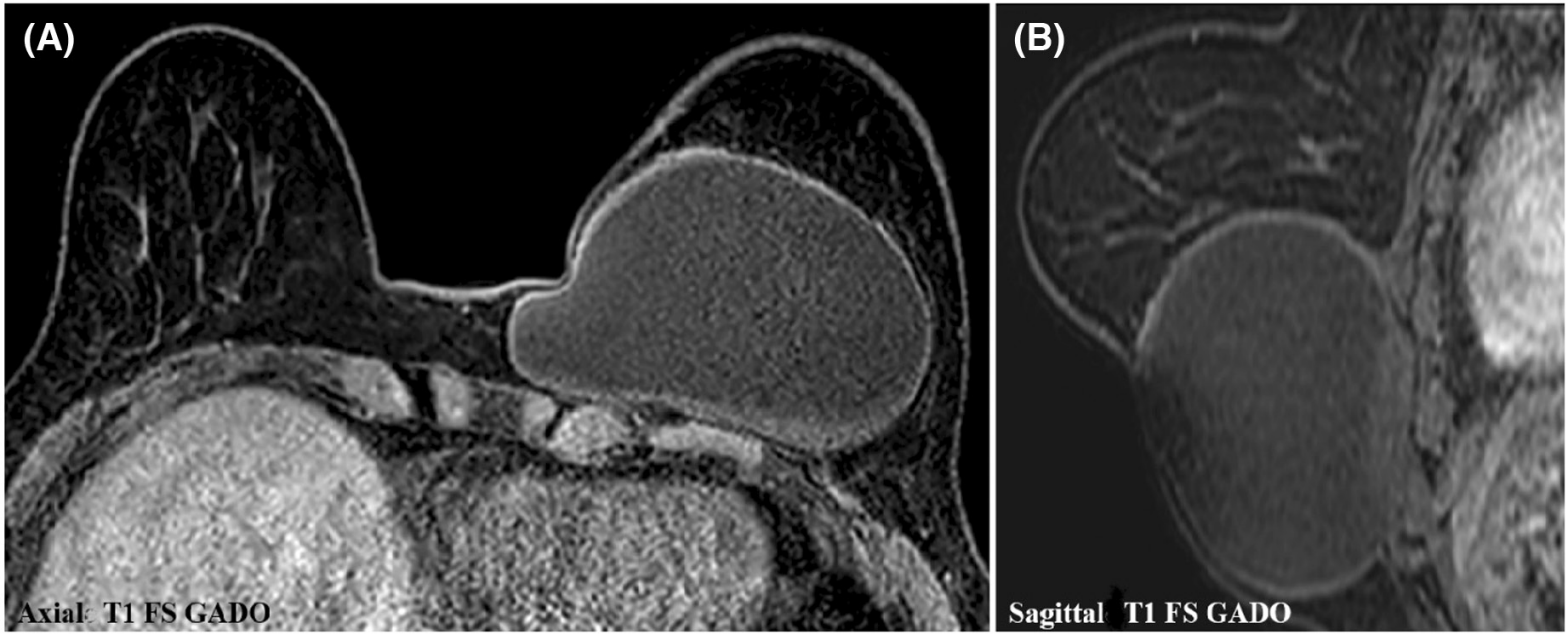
Axial *(A)* and sagittal *(B)* contrast‐enhanced T1‐weighted fat‐suppressed MR images show a regular enhancement of the capsular wall
